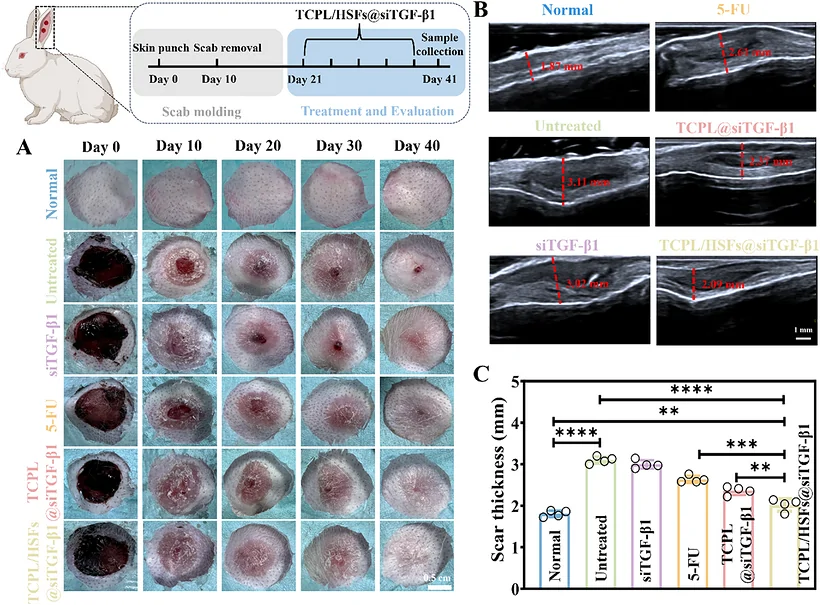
Establishment of the rabbit ear hypertrophic scar model and subsequent treatments. (A) Representative photographs of hypertrophic scar sites on rabbit ears at predetermined time points after various treatments. (B) Ultrasound imaging of hypertrophic scar sites on rabbit ears from each group at day 40 (scale bar = 1 mm). (C) Quantitative analysis of skin and scar thickness in the normal skin and experimental groups (n = 4, biological replicates). *P* < 0.01; P* < 0.001; *P* < 0.0001.
